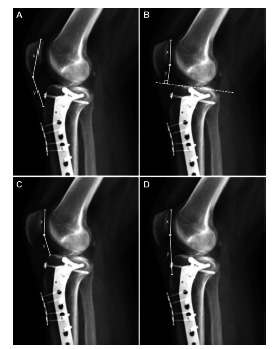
Sample measurements of patellar height index values are seen on the X-ray of the patient who underwent lateral plate due to tibial plateau fracture. A second plate was applied for fixation of the anterior fragment of the patient’s tibia metaphysis. In terms of the measurements: A) Insall-Salvati index; B) Blackburne-Peel index; C) Caton-Deschamps index; D) Modified Insall-Salvati index
